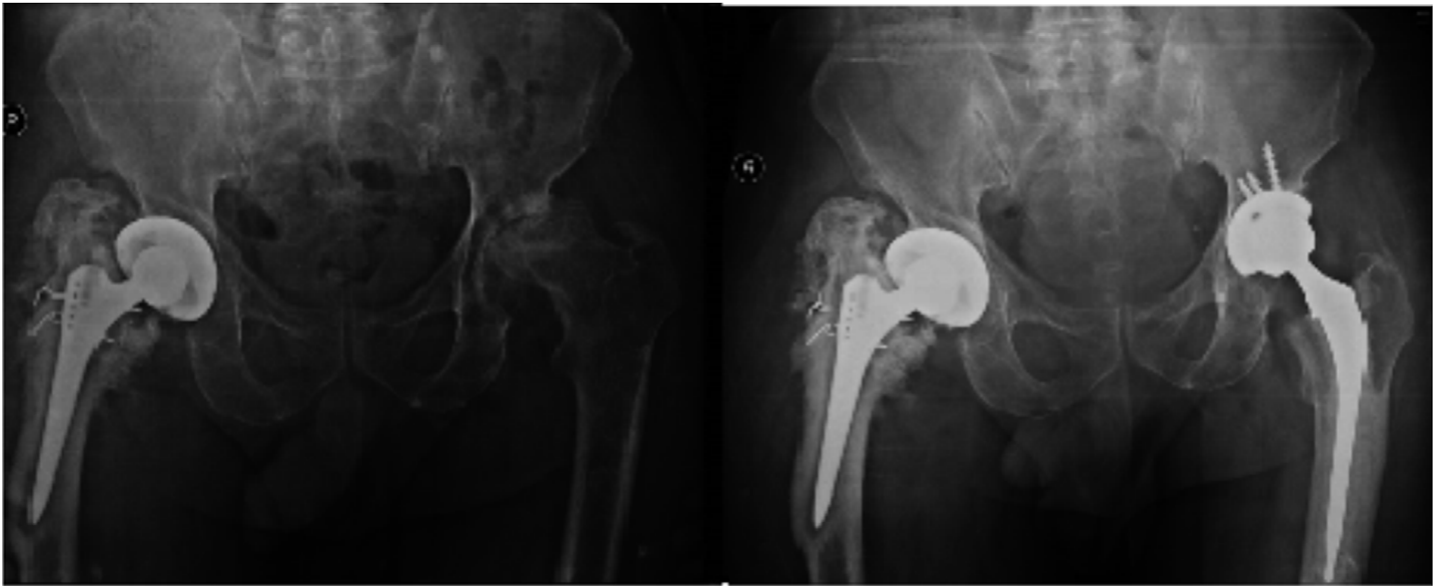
Preoperative and postoperative X-ray images for left total hip arthroplasty performed for severe hip osteoarthrosis. The loose, infected right hip prosthesis is evident.
